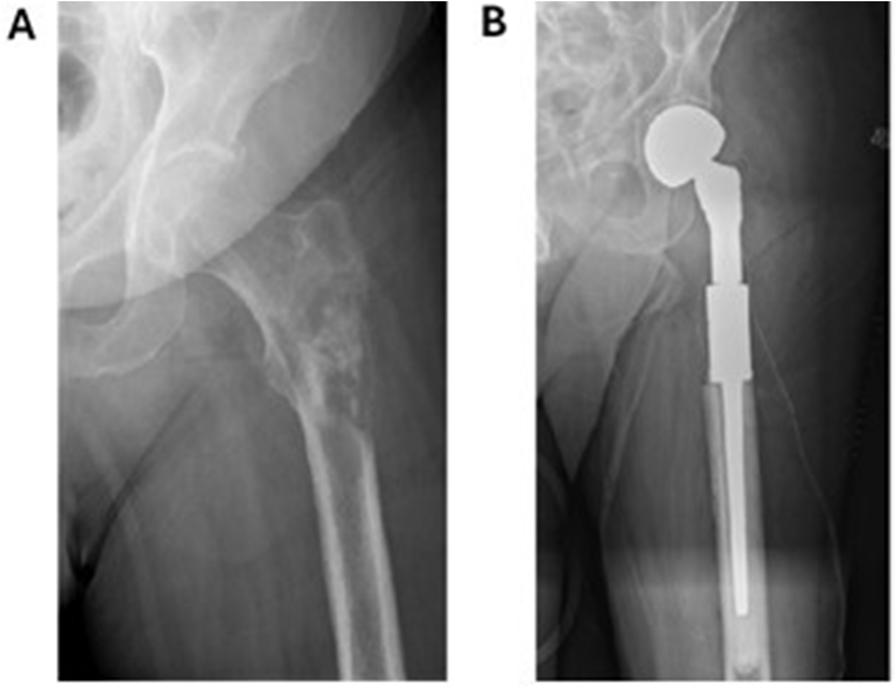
Preoperative and postoperative imaging of a 65-year-old patient with generalized prostate cancer treated at KS. A 65-year-old man with a 7-year history of prostate cancer and known skeletal and visceral mestastases. He represents with increasing pain in the femur. Radiographs demonstrate a pathological fracture through a known femoral metastasis. B The patient underwent resection of the proximal femur and reconstruction with a cemented proximal femoral megaprosthesis
